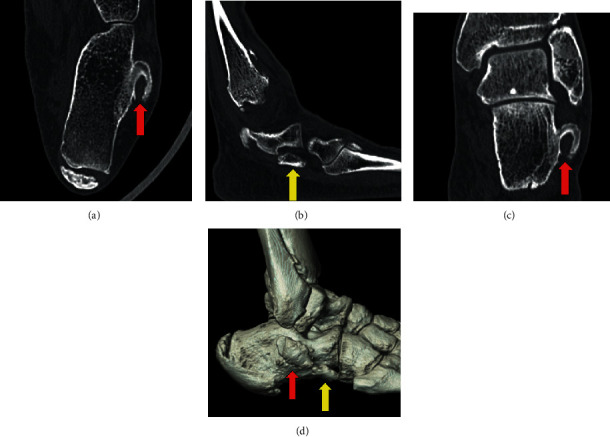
CT: Axial image (a), sagittal image (b), coronal image (c), and 3D image (d). Hypertrophic peroneal tubercle, a bony overgrowth surrounding a peroneal tendon (red arrow). Os peroneum, an ossicle on the outer anterior side of the calcaneus (yellow arrow).
